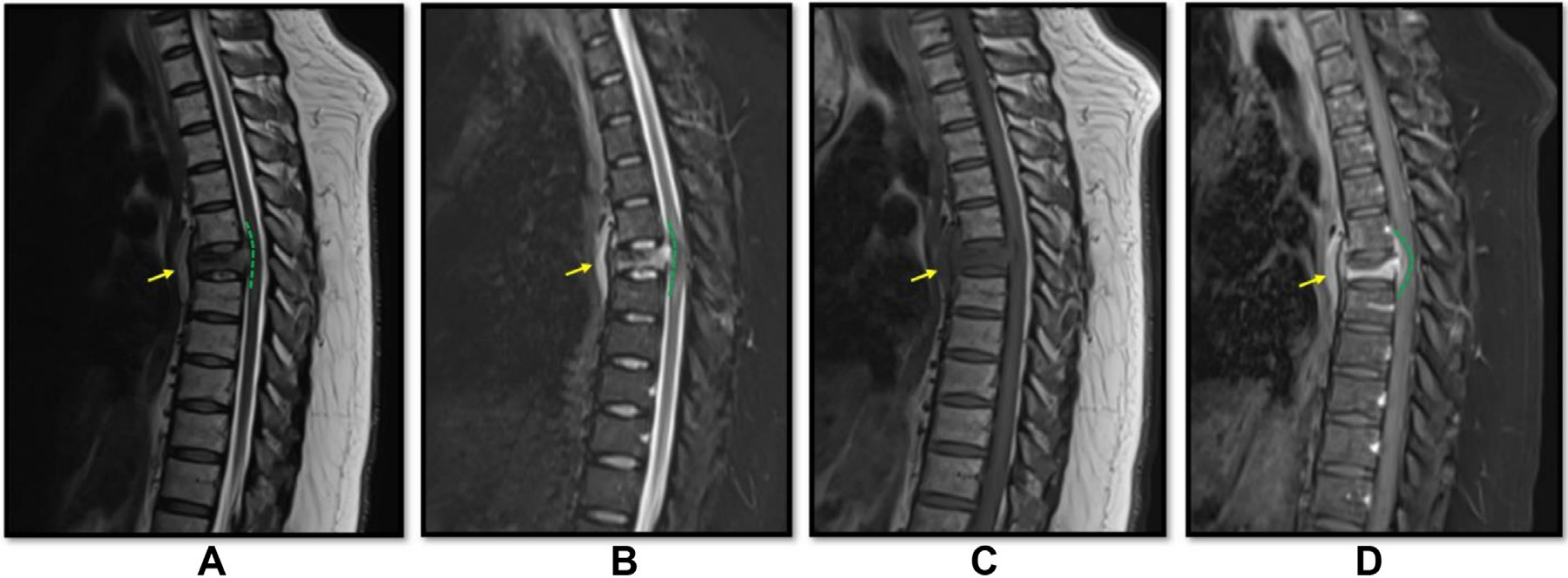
Selected images of multiplanar multisequence MRI of thoracolumbar spine with intravenous contrast. Sagittal planes: (A) T2 (B) STIR (C) T1 (D) T1 (D) T1 post contrast. There is T6 vertebral body collapse with underlying abnormal marrow signal of T1 low and T2/STIR bright signal and heterogenous postcontrast enhancement. Element of posterior retropulsion as well as associated prevertebral, paravertebral and retrovertebral intraspinal ventral epidural enhancing soft tissue component at the same T5-T6 level (green dotted line) with moderate cord compression.
